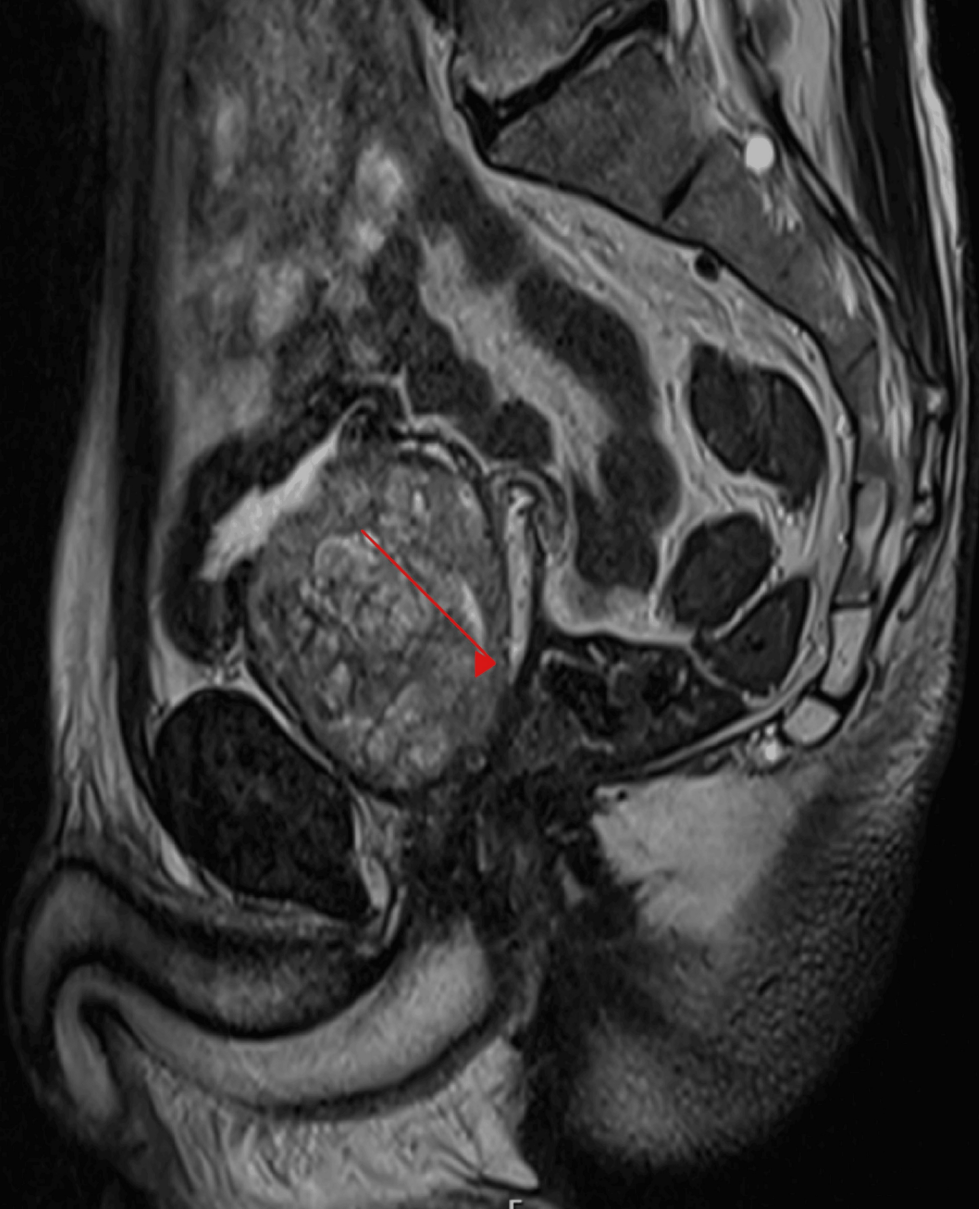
Pre-operative MRI sagittal view showing enlarged prostate (red arrow)
